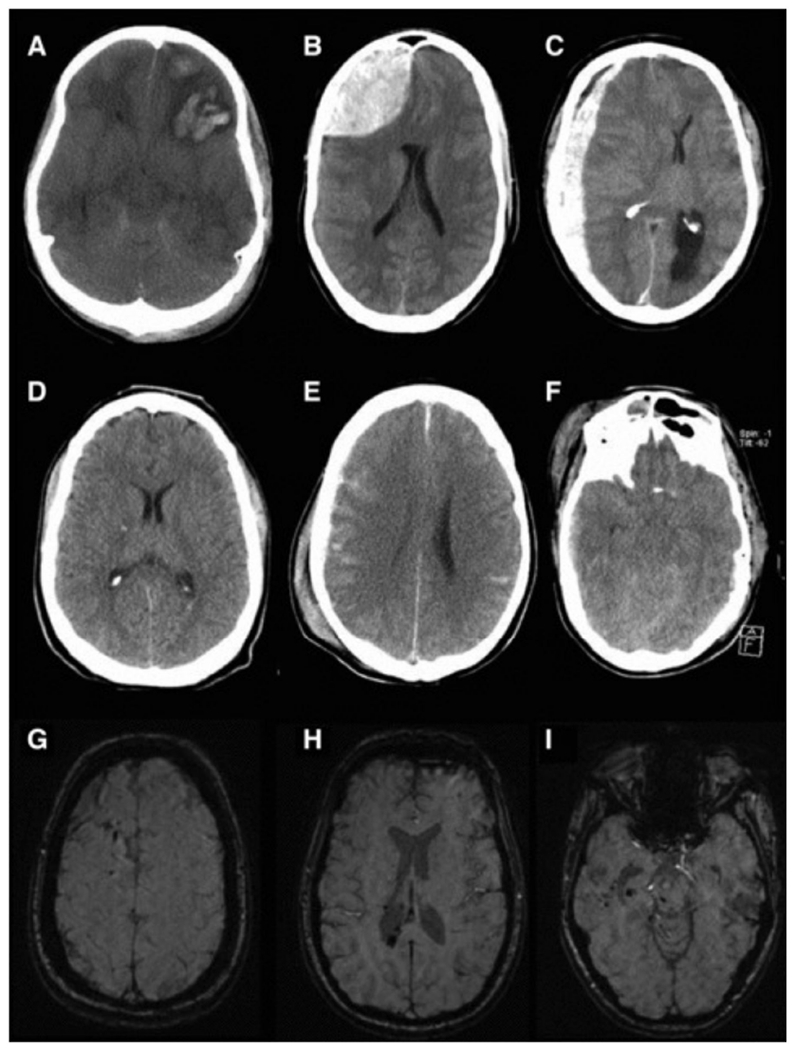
Examples of structural changes following focal and diffuse TBI represented by CT imaging (A–F) and MRI (G–I). (A–C) are of CT images following focal injuries, indicated by the presence of a focal contusion in (A), as well as hematomas in (B,C). Figures (D–F) are of CT images following diffuse injuries, indicated by hemorrhages in (D,E), and diffuse swelling in (F). Images (G–I) are of susceptibility weighted MRI images of one patient presenting with DAI indicated by hemorrhaging in different regions of the brain. Reproduced with permission from [27].
